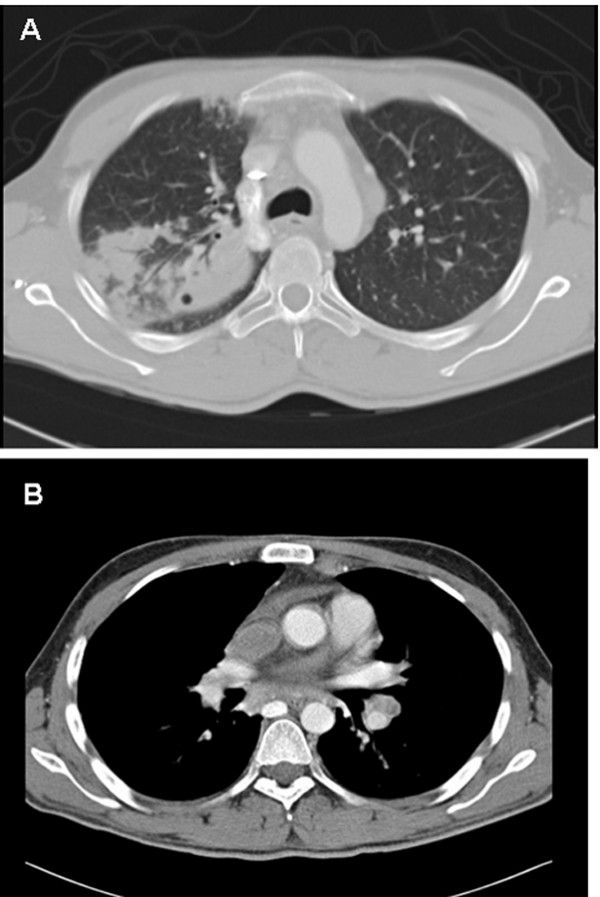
Chest CT scan showed right lung consolidation (A), with enlarged mediastinal lymph nodes (B).
